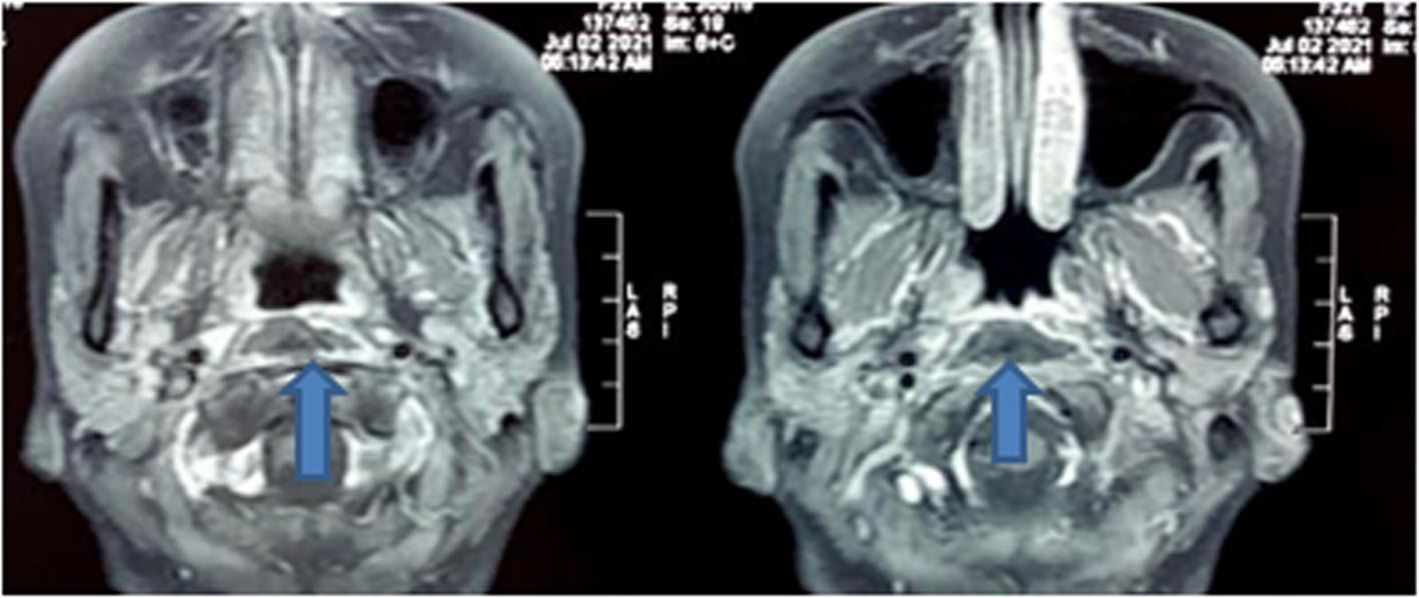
T1 contrast MRI image (Axial section) showing retropharyngeal abscess with no contrast uptake
